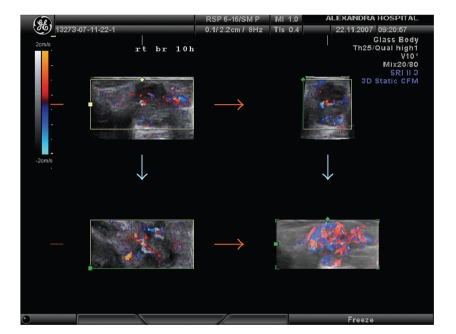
3D Power Doppler imaging of a malignant tumor with chaotic vessels' architecture and complex branching pattern.
